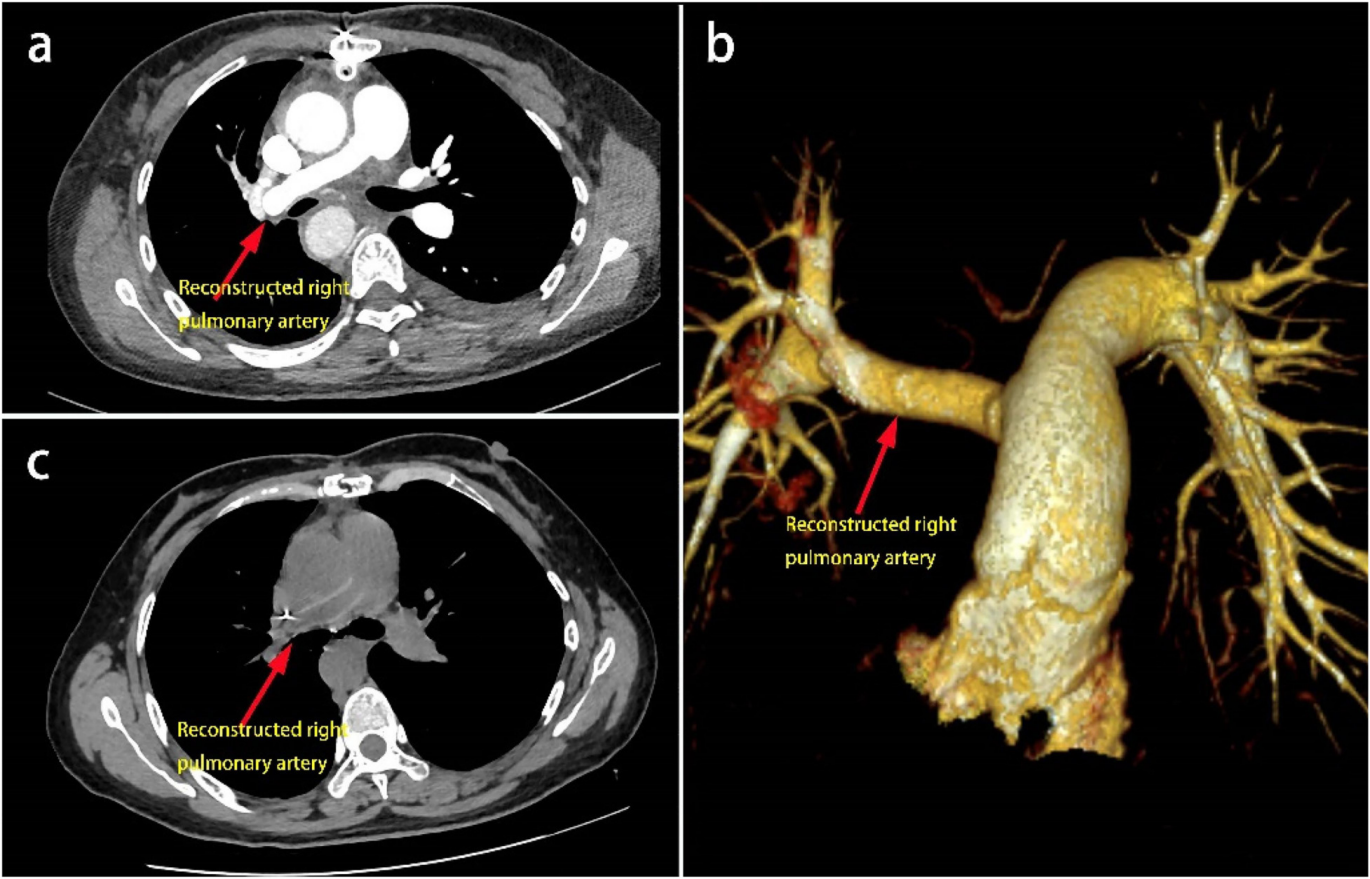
(a,b) follow-up chest CTA demonstrates patency of the artificial vascular graft with no significant filling defect observed; (c) Two-month postoperative chest CT reveals that the right pulmonary artery trunk is narrower than the contralateral side, but no obvious filling defect is seen within it.
